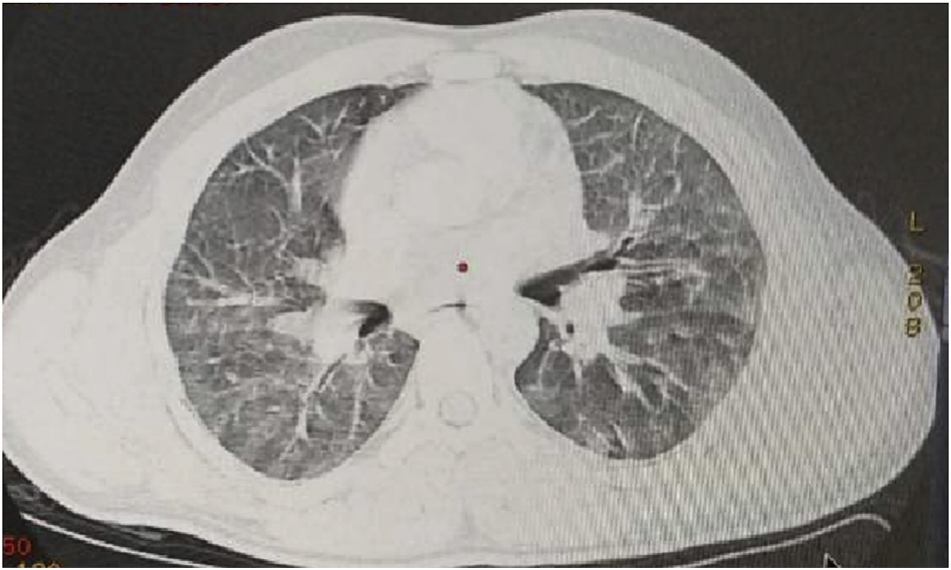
Computed tomography scan of a 25-year-old male subjected to blunt chest trauma revealing bilateral pulmonary contusion.
